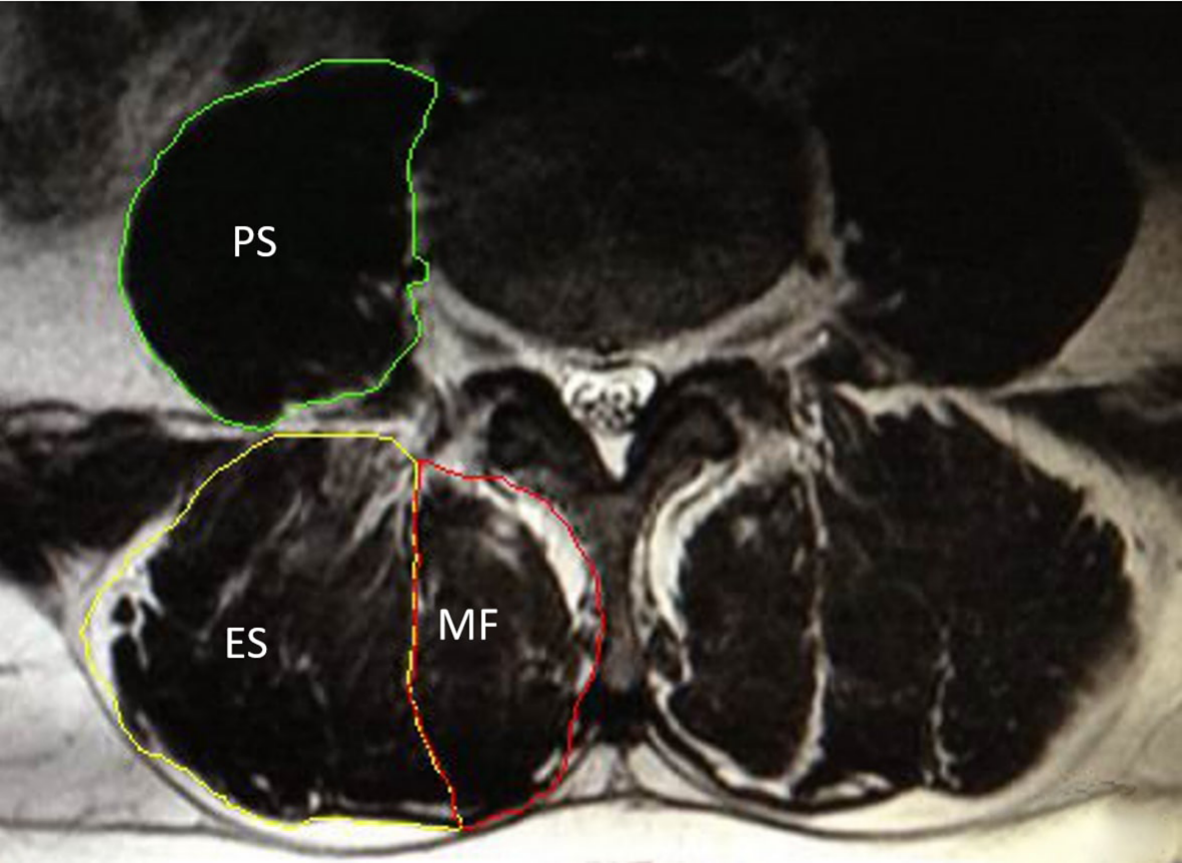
Axial MRI at the lumbar level showing segmentation of paraspinal muscles: psoas major (PS), erector spinae (ES), and multifidus (MF).
